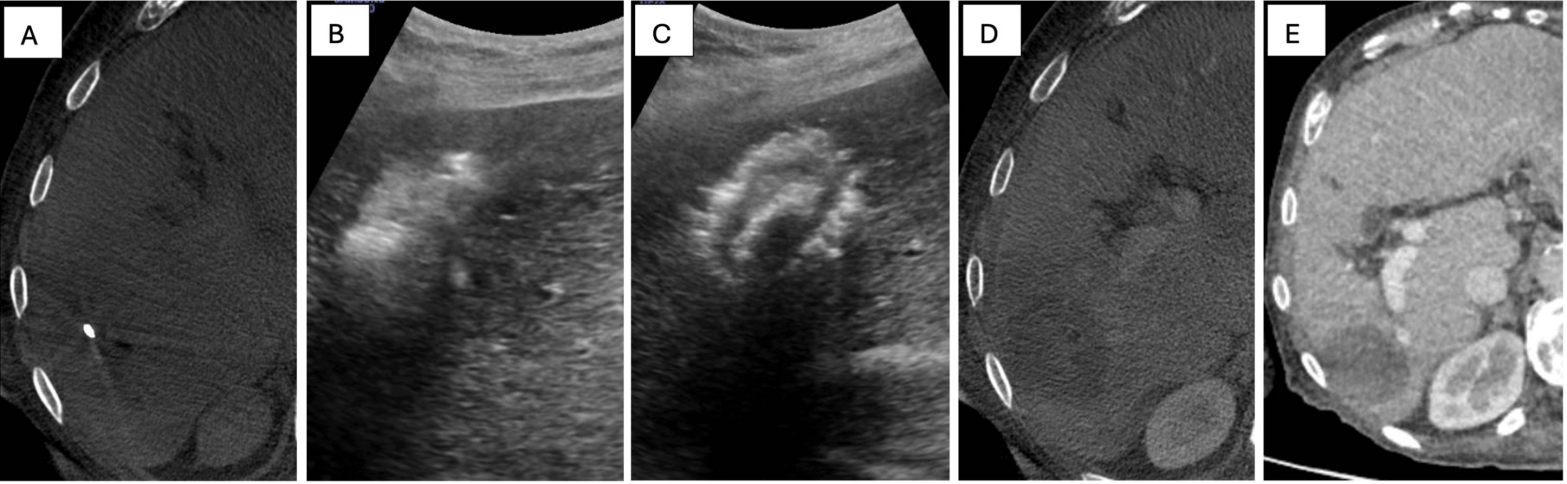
MWA of primary HCC: appearance during and after ablation Intra-procedural and post-procedure appearance of the same HCC lesion as in Figure 2 is shown with the probe at the centre of the lesion on both Dyna-CT and ultrasound. The radio-dense thermal probe is shown within the HCC lesion on Dyna-CT (A). The thermal probe can be seen within the lesion on ultrasound as the microwave is applied producing hyperechoic gas (B). Appearance immediately following ablation is demonstrated on ultrasound with hyperechoic gas at the site of ablation (C). Contrast Dyna-CT shows the immediate post-ablation appearance and confirms adequate coverage (D). Conventional contrast CT demonstrates successful ablation with no recurrence at three months (E). MWA: microwave ablation; HCC: hepatocellular carcinoma; CT: computed tomography; Dyna-CT: dynamic volume computed tomography
